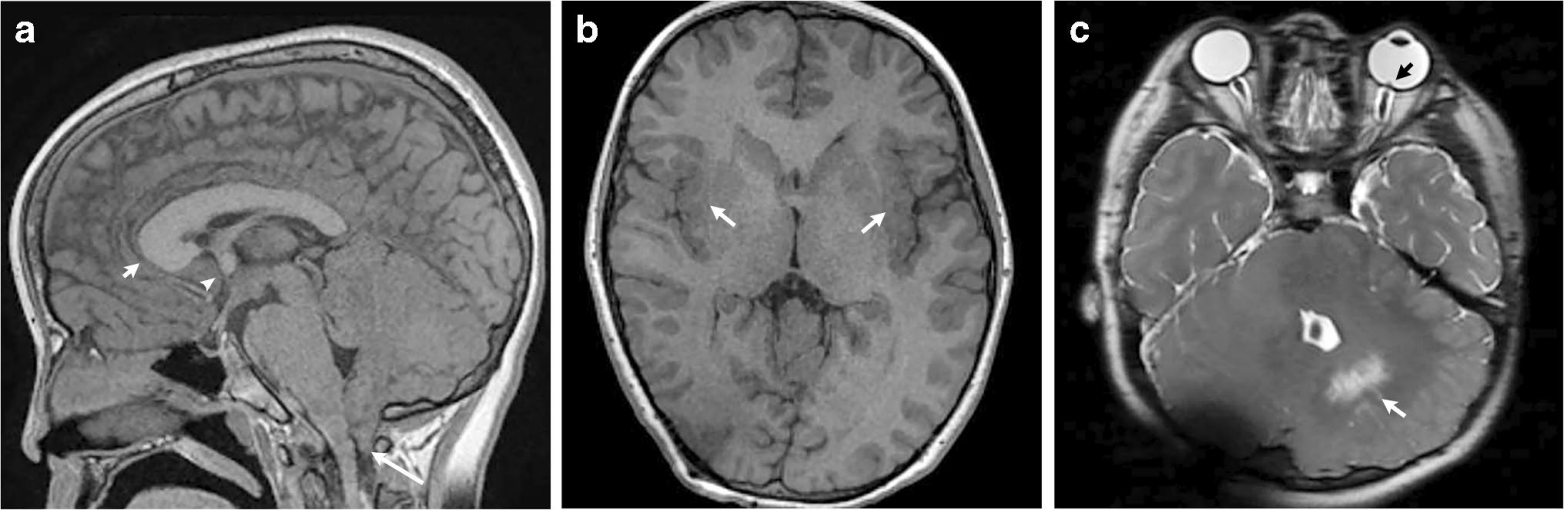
Cowden syndrome in a 10-year-old boy with genetically confirmed *PTEN* mutation. a A sagittal spoiled gradient recalled echo (SPGR) image shows an enlarged corpus callosum that is greatest at the anterior aspect (*short arrow*), along with an enlarged anterior commissure (*arrowhead*). The cerebellum is enlarged, and there is tonsillar ectopia (*long arrow*). b An axial SPGR image shows bilateral perisylvian polymicrogyria (*arrows*). Note the increased volume of the white matter. c An axial T2-weighted image shows marked enlargement of the cerebellar hemispheres with a dysplastic appearance and hyperintense signal on the left (*white arrow*), which likely represents Lhermitte-Duclos disease although it is not classic in appearance. The orbital globes show hypertelorism and papilledema (*black arrow*) from increased intracranial pressure. Susceptibility artifact is noted in the right posterior fossa from a ventriculoperitoneal shunt catheter
